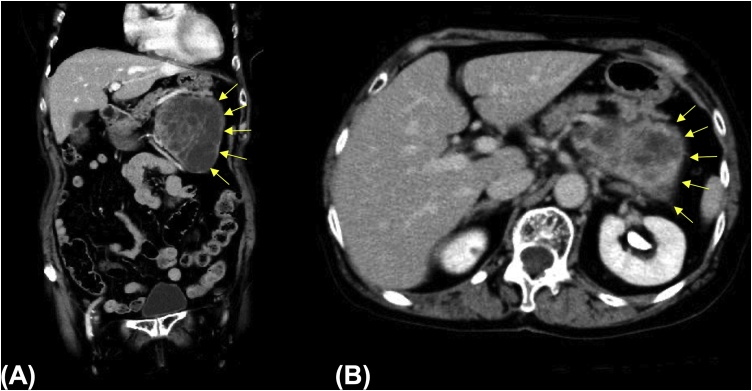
Enhanced computed tomography image of the abdomen. Coronal (A) and (B) images showing a giant tumor measuring 15 cm along the greatest dimension.
